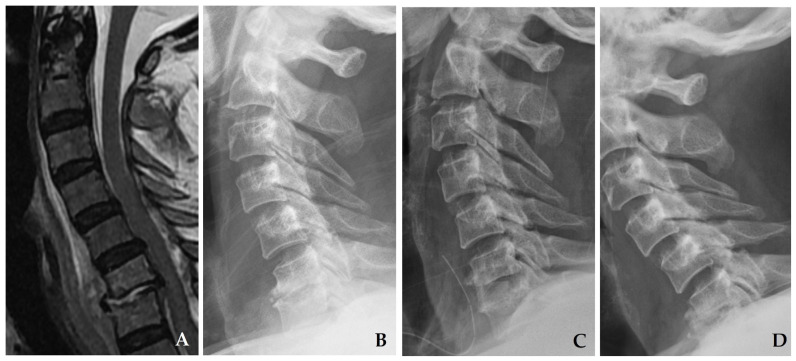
Initial magnetic resonance imaging (A) and plain radiograph (B) demonstrate disc space narrowing at C6–C7 with a retropharyngeal abscess. The patient underwent C6–C7 discectomy with autograft without anterior plating (C); however, collapse of the autograft and development of kyphosis were observed at two months postoperatively (D).
